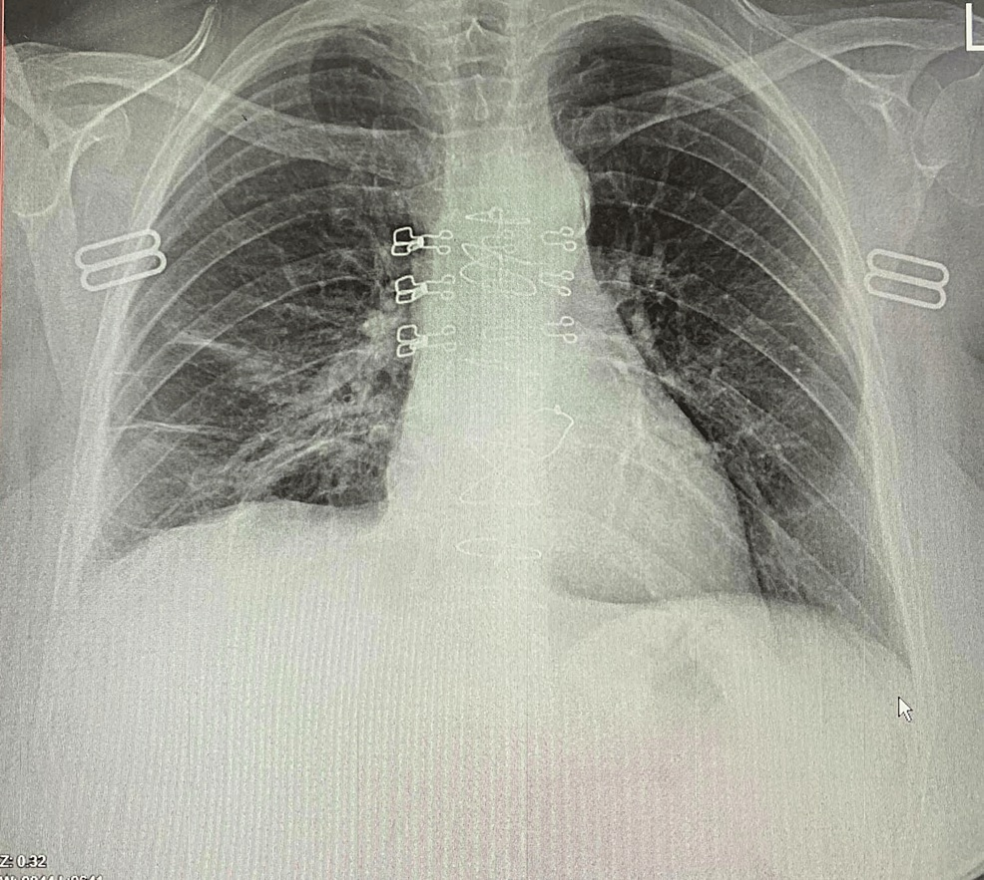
Chest X-ray of the patient on follow-up
